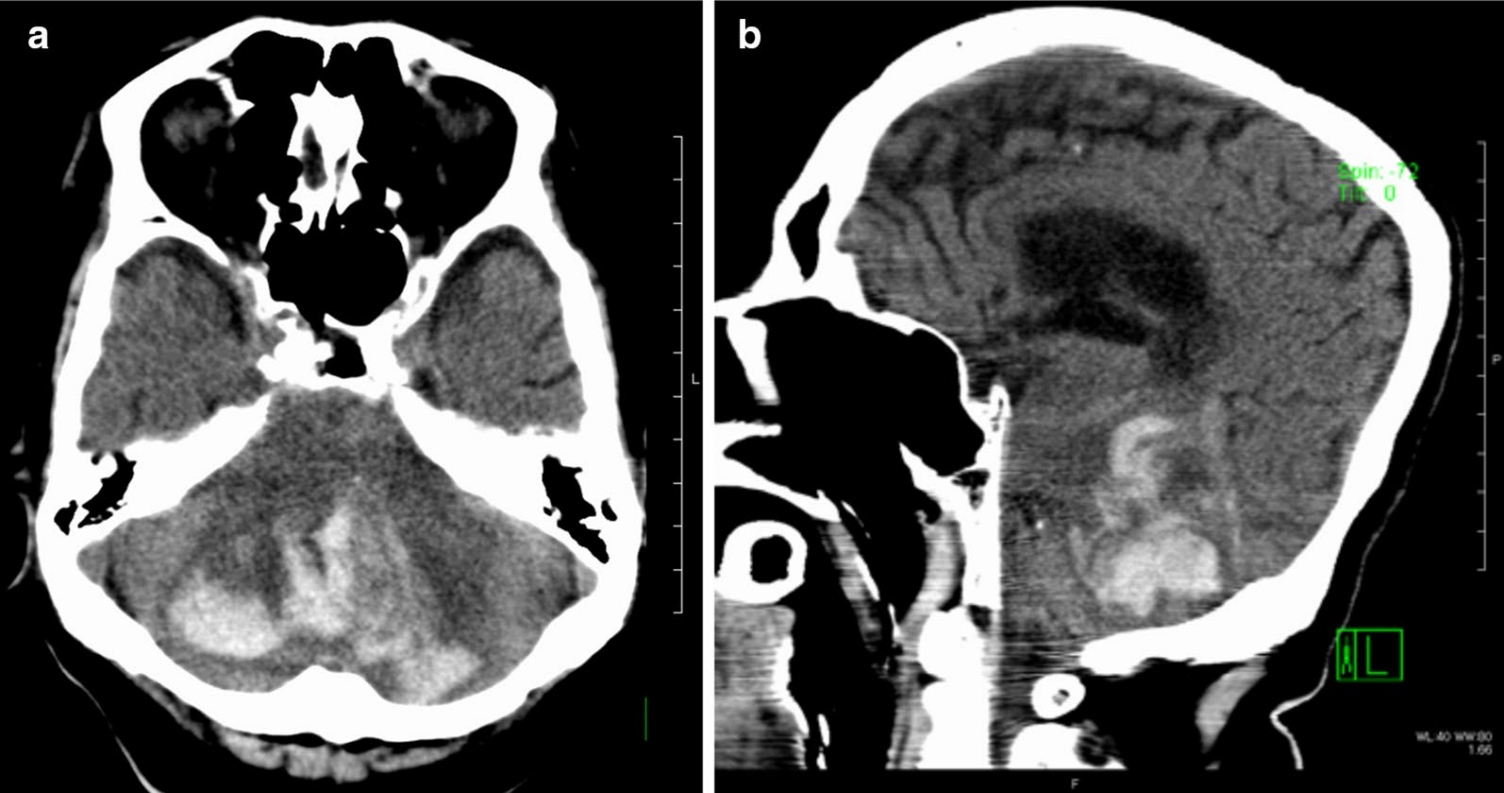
Brain computed tomography (CT) images of case 1. Brain CT revealed cerebellar bleeding on POD 27
